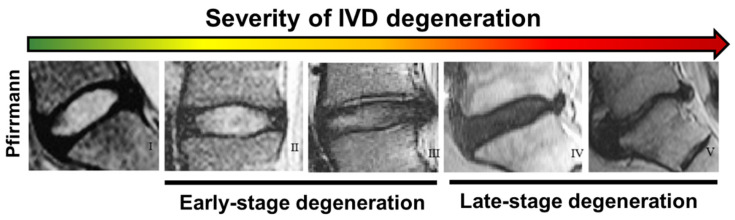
Comparison between different stages of intervertebral disc degeneration (I to V, left to right) according to the Pfirrmann grade scale based on MRI images. Reprinted and adapted with permission from Ref. [103]. Copyright 2022, Elsevier.
